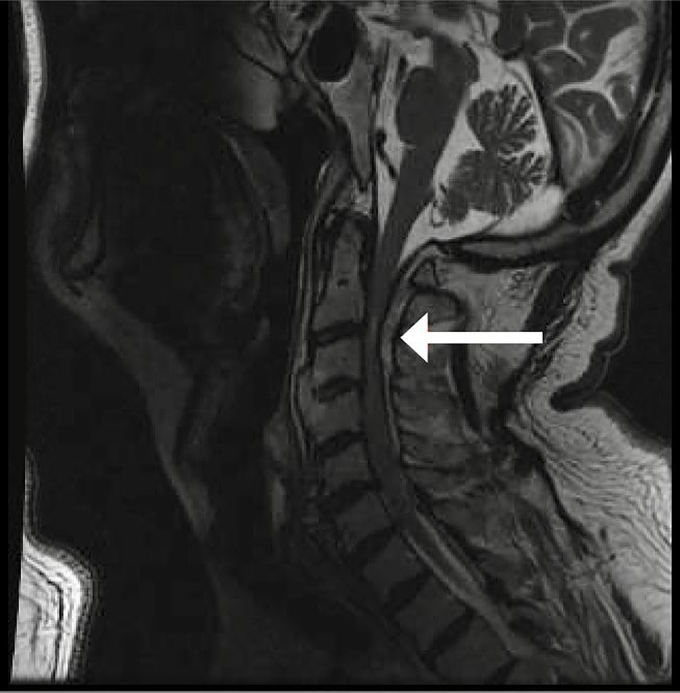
Magnetic resonance imaging (T2 weighted) in sagittal view demonstrating spontaneous spinal epidural hematoma from the foramen magnum to cervical spine level 7 as indicated by the arrow.
